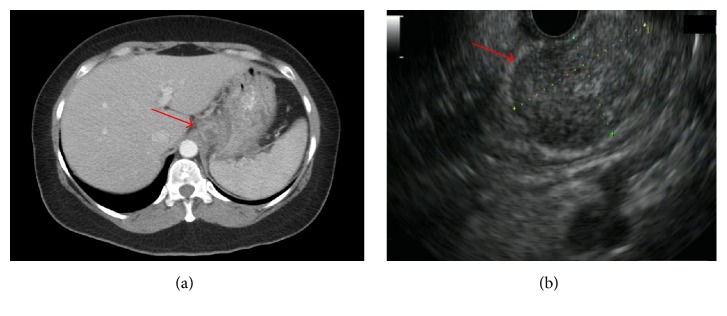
Computer tomography (CT) scan revealing a 1.2 × 1.0 cm low attenuation lesion adjacent to the gastric fundus ((a) red arrow); the lesion was also visualized on endoscopic ultrasound ((b) red arrow).
